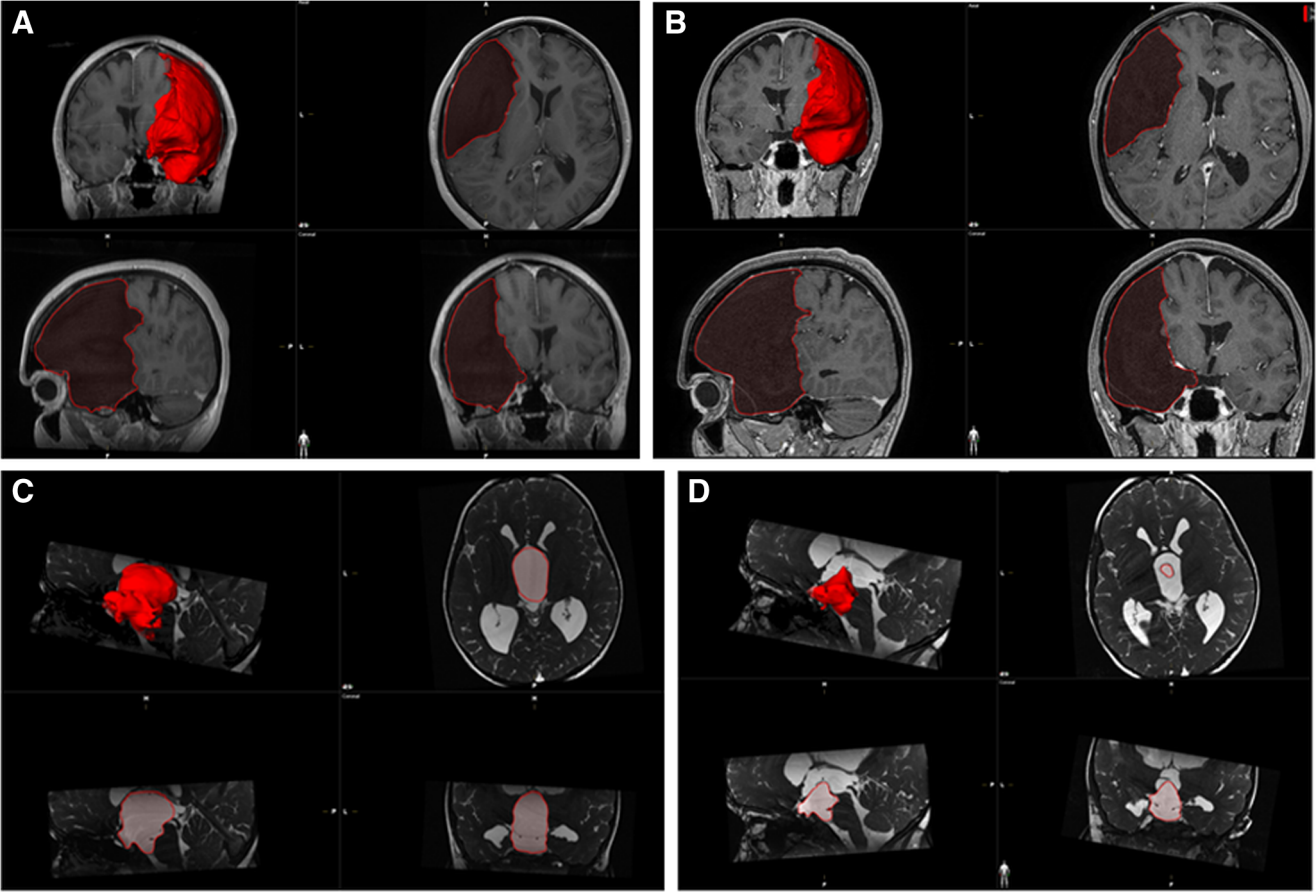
Images showing the method for three-dimensional cyst visualization and calculation of its volume with application of the the SmartBrush software (Brainlab, Germany). The 3D T1-weighted contrast enhanced images a (preoperative) and b (postoperative) concern patient 8. In this case, there was a small but detectable decrease of the cyst volume postoperatively from 269.9 to 256.9 cm^3^ as well as a minimal decrease of the midline shift from 6 to 5.5 mm. This patient had persistent headache in the long-term 3-year follow-up. The T2-weighted steady-state (3D-DRIVE) images c (preoperative) and d (postoperative) demonstrate the radiological results from treatment of patent 14. Postoperatively, there was a substantial decrease of the cyst volume (from 43.4 to 18.2 cm^3^) as well as evident flow void through the cyst 1 year after the surgery. There was no midline shift both before and after surgery. The patient improved neurologically, presenting no symptoms of HCP and minimal somatotropine pituitary insufficiency, controlled with growth hormone substitution
